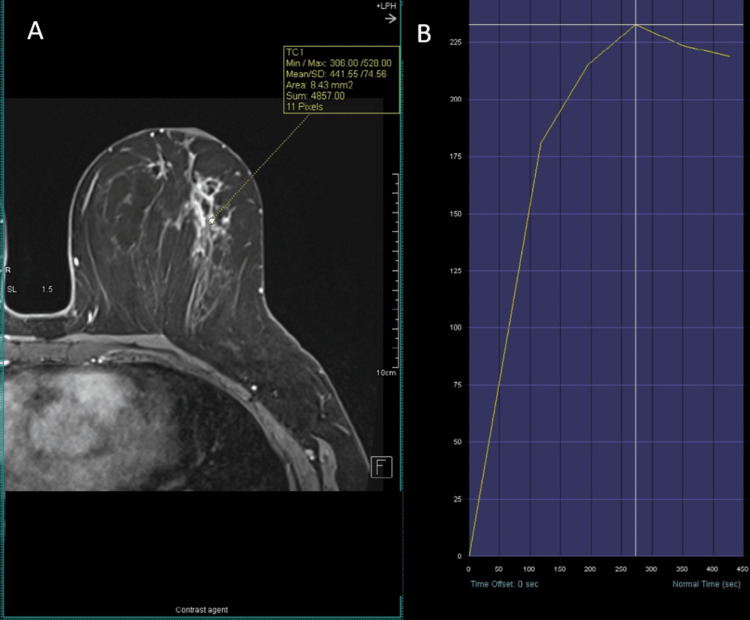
Breast MRI (DCE) A) DCE MRI with a representative region of interest for kinetic analysis. B) Type III (washout) kinetic curve. MRI: magnetic resonance imaging; DCE: dynamic contrast-enhanced
